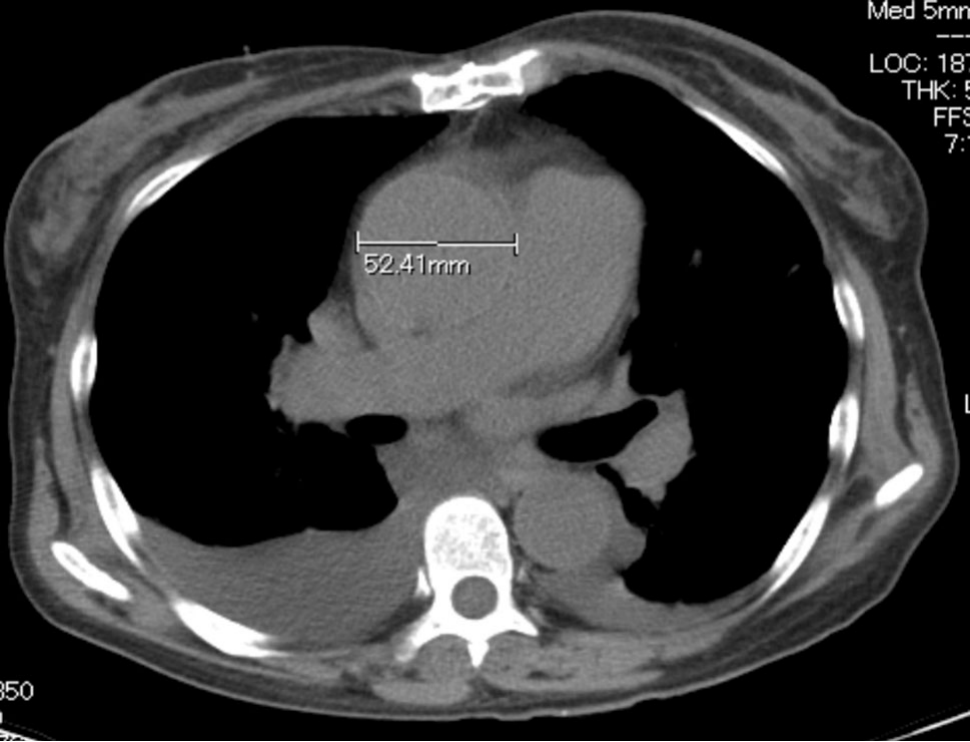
Preoperative computed tomography showed a dilated ascending aorta
